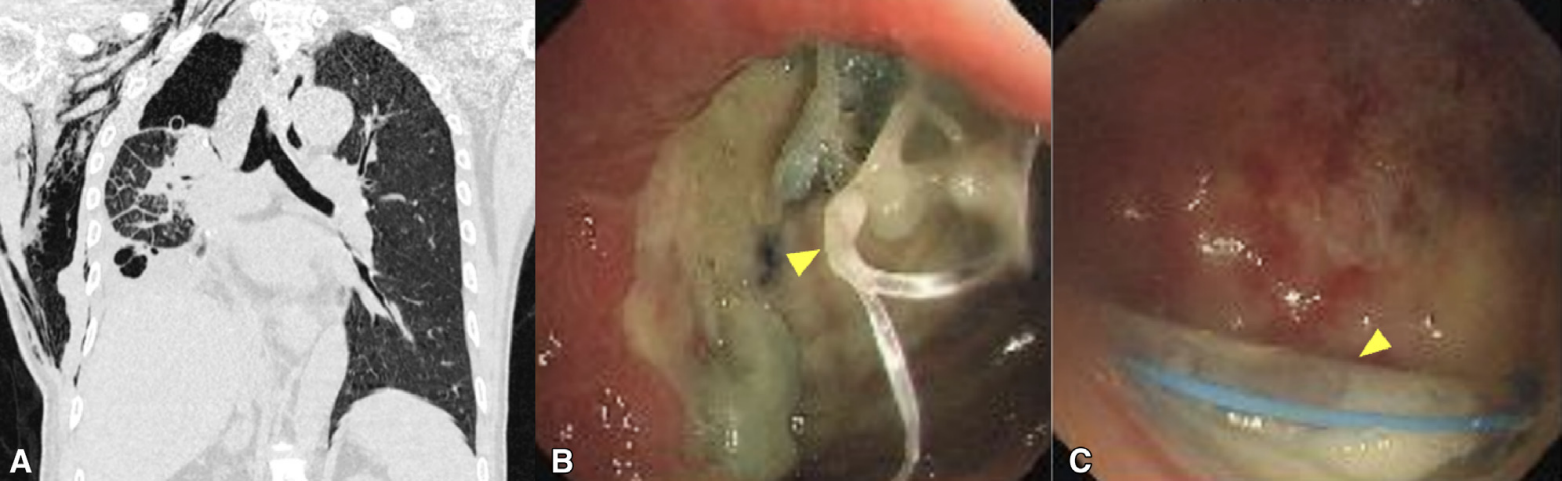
A, Computed tomography image showing a right-sided hydropneumothorax and subcutaneous emphysema. B, A fistula at the right main bronchial anastomosis with a broken suture (*arrow*). C, The right thoracic cavity and drainage tube (*arrow*) are visible through the fistula.
